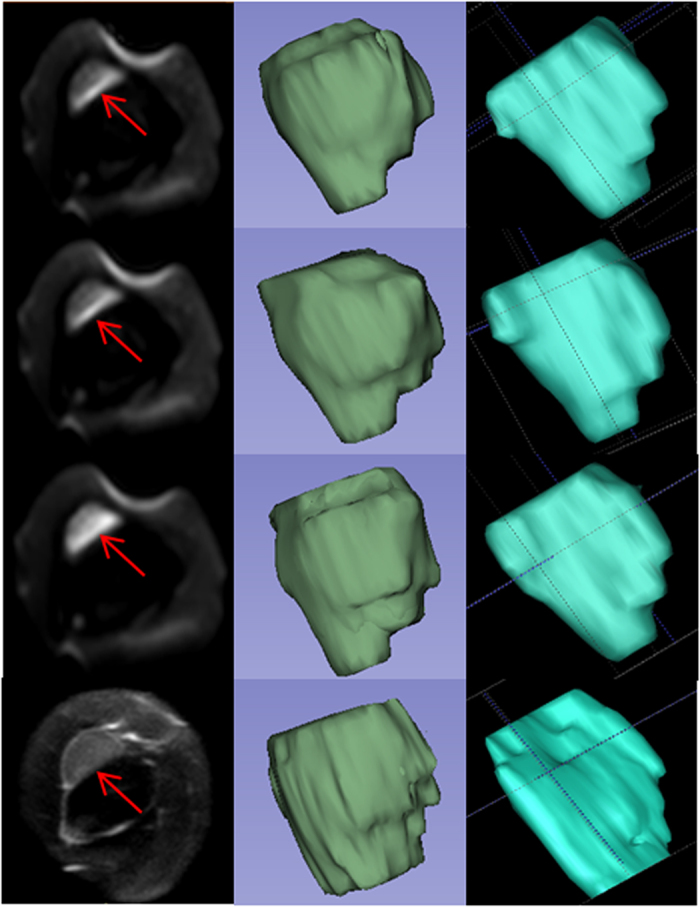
MRI-based tumour volume segmentations with the semiautomatic and manual segmentation methods. The leftmost images present the same subcutaneous tumour (red arrows) on an axial slice: b = 0 s/mm^2^ DWI (uppermost), b = 20 s/mm^2^ DWI (second image from the top), b = 800 s/mm^2^ DWI (third image from the top), and mMRI MRI (lowest). Moreover, comparison of total tumours in corresponding sequences obtained from semiautomatic segmentation by 3D Slicer (green, middle images) and manual segmentation by ITK (blue, rightmost images) are presented.
